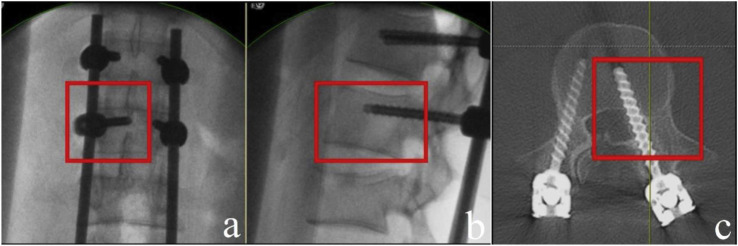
a) Intra-operative fluoroscopy image showing the left lumbar level 2 pedicle screw tip touching the midline on a PA view; b) Screw length spanning up to 50% of the vertebral body in the lateral view; c) Post-operative axial CT image showing a major medial breach of the pedicle.
